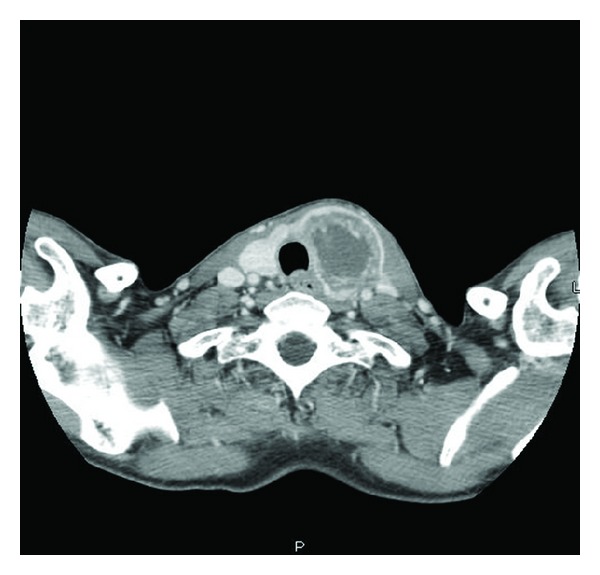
Neck CT: A reveals a large heterogeneous mass that has replaced the left lobe of the thyroid gland.
